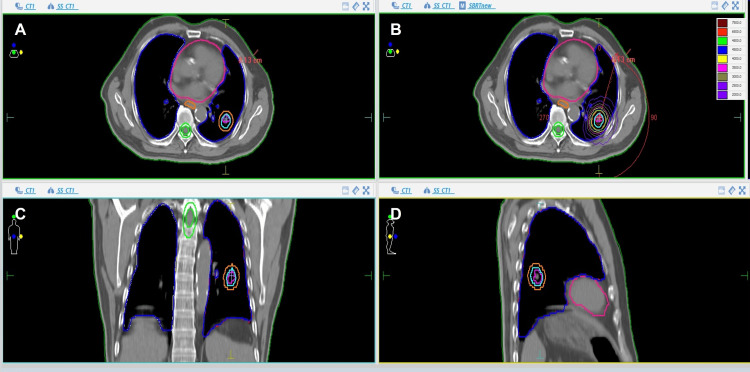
Target delineation and radiotherapy plan of 4DCT-SBRT (A, C, D) Axial, coronal, and sagittal views showing target volume delineation. The blue line indicates ITV, and the orange line indicates PTV. (B) VMAT treatment plan. ITV: Internal target volume; PTV: Planning target volume; VMAT: Volumetric modulated arc therapy; 4DCT-SBRT: Four-dimensional computed tomography-guided linear accelerator-based stereotactic body radiation therapy
